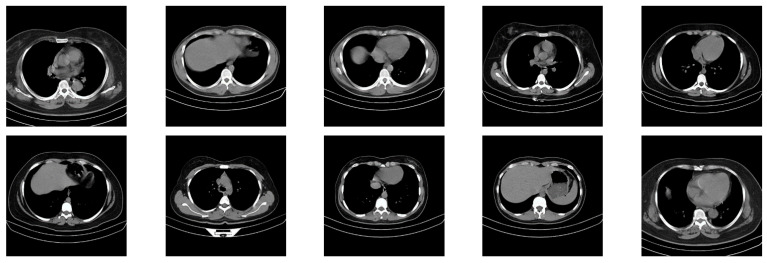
CT images used in the proposed method.
